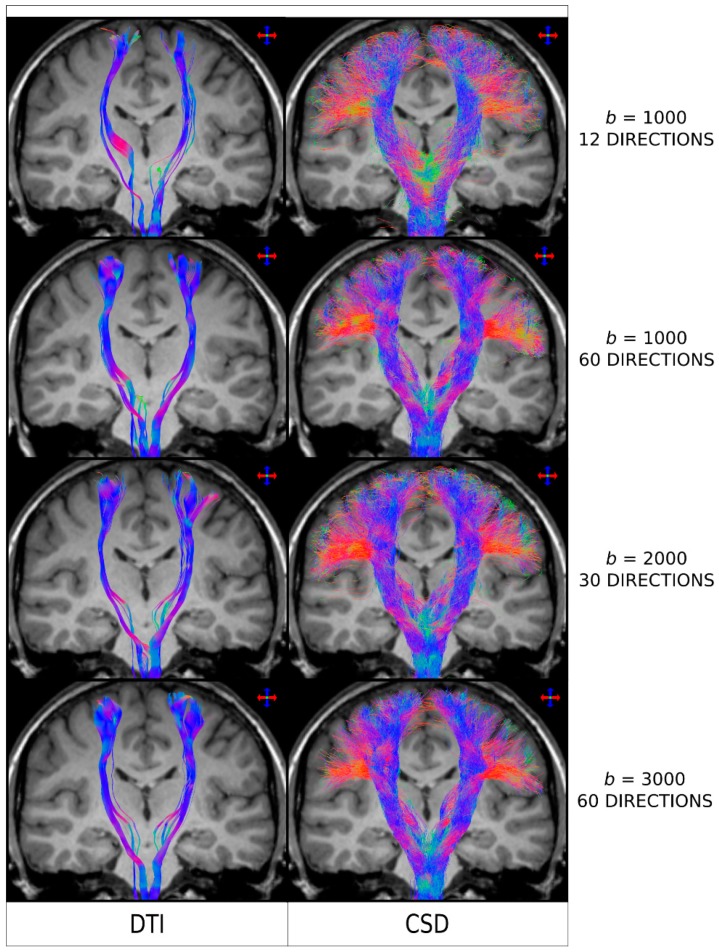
Fibre-tracking results on a healthy human subject obtained using a seed region in the brainstem and target regions in the sensorimotor cortices. Coronal T1-weighted images overlaid with tractography results using DTI combined with a deterministic algorithm (left column) and CSD combined with a probabilistic algorithm (right column), for a range of diffusion MRI protocols (as indicated by the b-value, in s/mm^2^, and number of directions). The colour-coding indicates the fibre orientation (same convention as in Figure 2). DTI: Diffusion Tensor Imaging; CSD: constrained spherical deconvolution. As clarified for the interpretation of the results from Figure 3, and based on the results reported in Figure 2, the general conclusions from this figure are also expected to be applicable to the case of combining DTI with probabilistic tracking. Figure modified from a figure previously published in [30], with permission of American Association of Neurological Surgeons, 2013.
